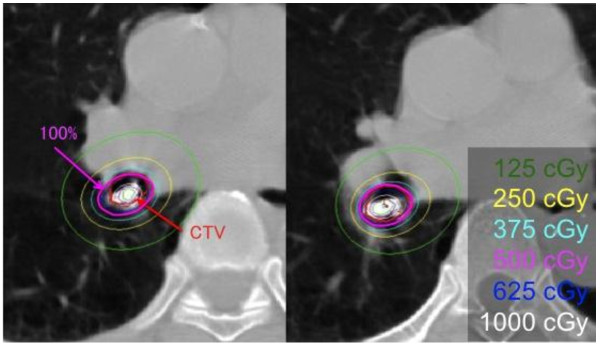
CT based dose distribution. A CT based dose distribution of the recent case. Red thick line is a delineated contour of clinical target volume (CTV) and magenta line indicates 100% of prescribed dose (5 Gy). 95% of the CTV receives more than 90% of the prescribed dose. The unit is cGy/fraction.
